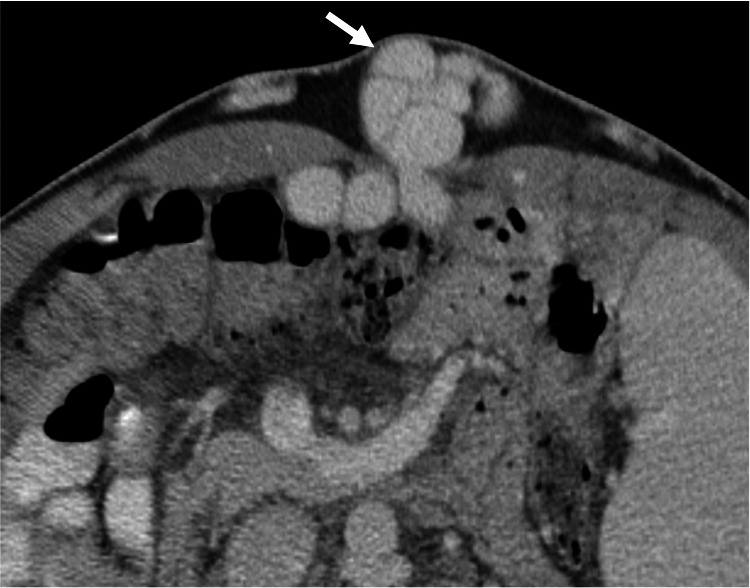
Axial contrast-enhanced abdominal CT scan in the venous phase showing abdominal wall varices Axial CT scan shows multiple dilated and tortuous venous structures with a diameter larger than 3 mm located in the anterior abdominal wall (white arrow); these findings are compatible with abdominal wall varices.
